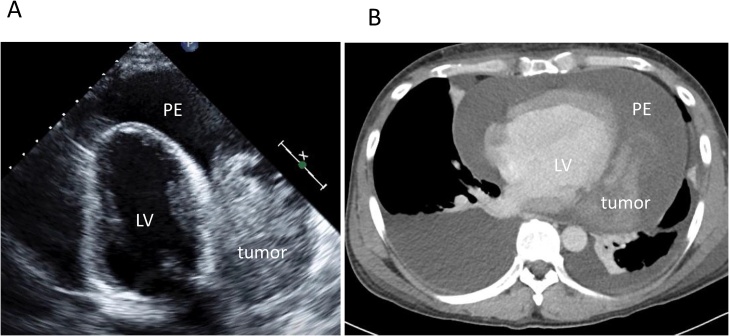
A. Transthoracic echocardiography findings. B. Computed tomography findings. Massive pericardial effusion and large tumor were detected. PE: pericardial effusion, LV: left ventricle.
